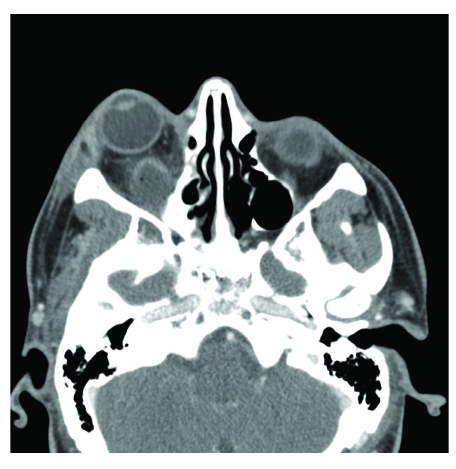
Repeat axial CT scan (hospital day 4) shows a right inferior orbital abscess
